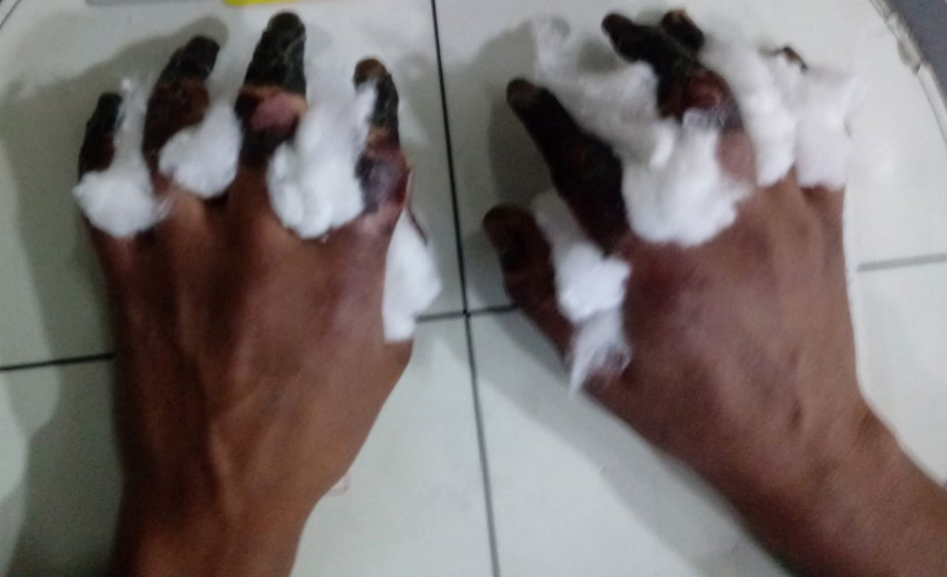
Physical examination demonstrated gangrene involving all digits of both hands, concluding a fourth-degree frostbite. Therefore, multiphase 99mTc-MDP bone scintigraphic imaging was performed to both hands
